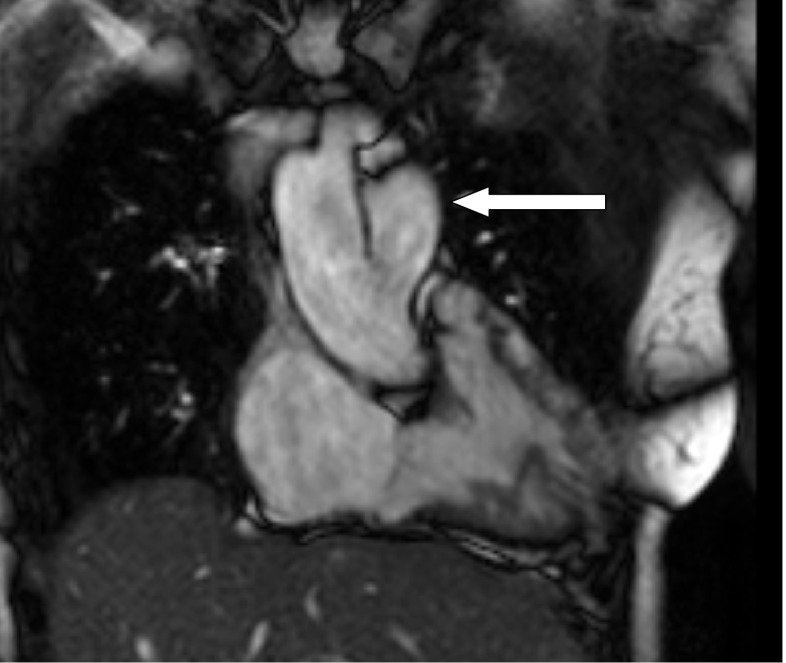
Magnetic resonance imaging showing the left pulmonary artery originating from the ascending aorta (*arrow*)
